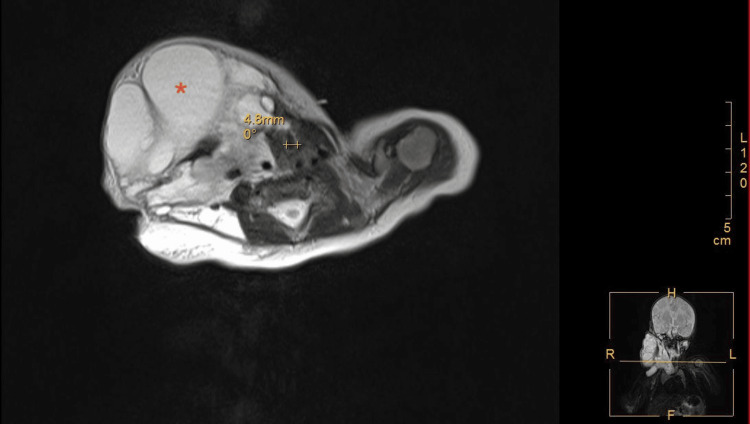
Magnetic resonance imaging of the macro-cystic hygroma（*） and the narrowed trachea（++） as measured
